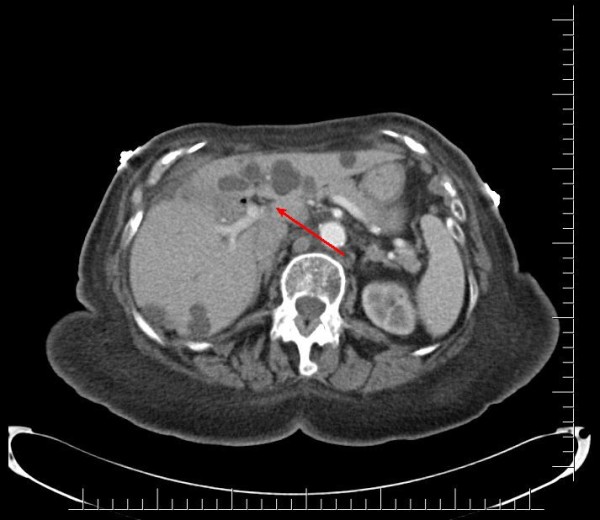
Axial computed tomography showing a hepatic cyst infiltrating upon the common hepatic duct and causing hepatic duct dilation.
